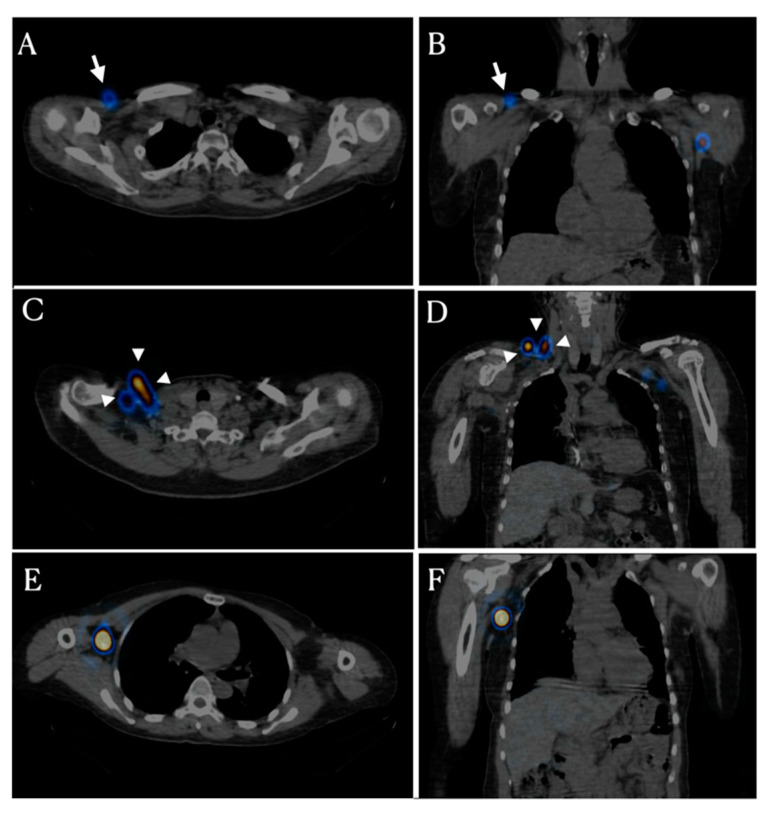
SPECT/CT findings of two types of bypass circulation. (A,B) The infraclavicular type shows radioactivity on the deltopectoral groove (arrow) below the right clavicle. (C,D) The supraclavicular type (arrowhead) shows radioactivity around the right clavicular fossa above the clavicle. (E,F) The right axillary lymph nodes are activated within the axillary fossa. However, there are no radioactivated axillary lymph nodes on left side following axillary lymph node dissection.
